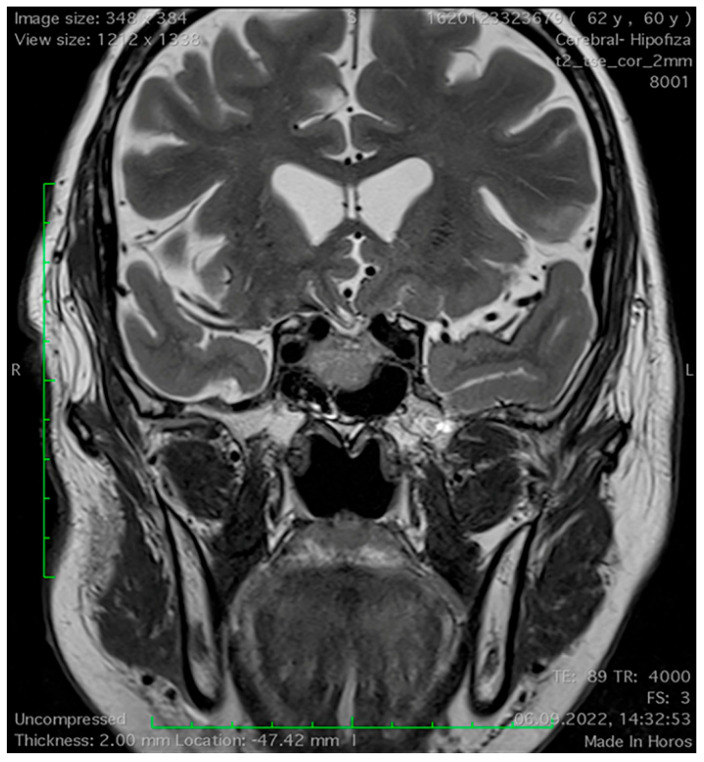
Mucosal thickening of the sphenoid sinus on a coronal T2−weighted magnetic resonance imaging section in a patient with pituitary adenoma.
